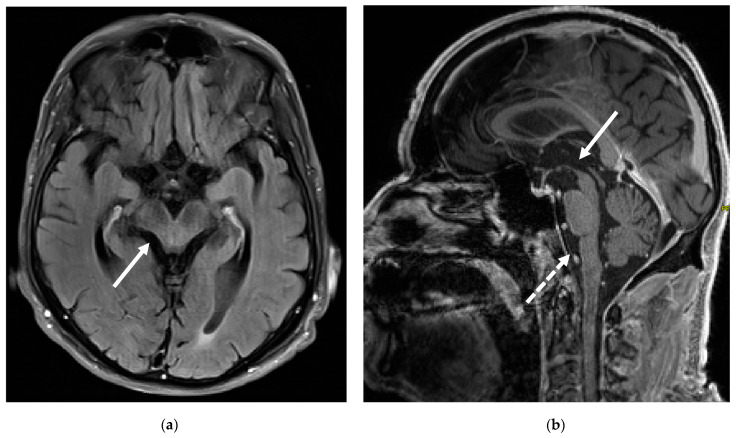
Cerebral MRI at follow-up four years after baseline MRI. (a) Axial FLAIR sequences showing midbrain atrophy (white solid arrow). (b) Sagittal contrast-enhanced T1-weighted sequences now demonstrating atrophy of the midbrain (white solid arrow) and the medulla oblongata (white dotted arrow).
